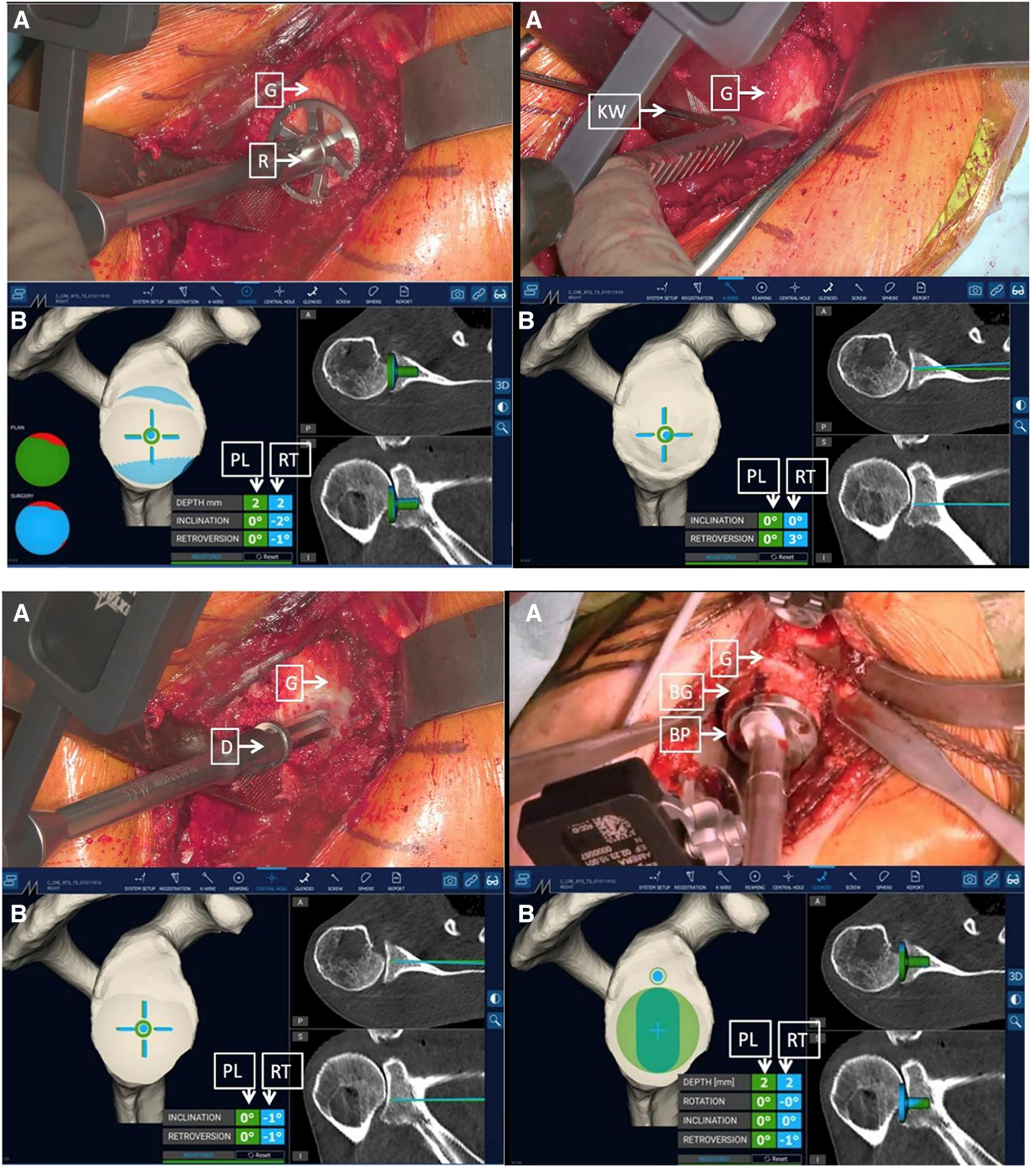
Surgeon's intraoperative field of view. The augmented reality navigation system provided planned (PL) and real-time (RT) pitch and yaw values. On the right side of the image, the two-dimensional CT scan images display the axial (top) and coronal (bottom) PL (green line) and RT (blue line) directions (D, drill; G, glenoid) (18).
